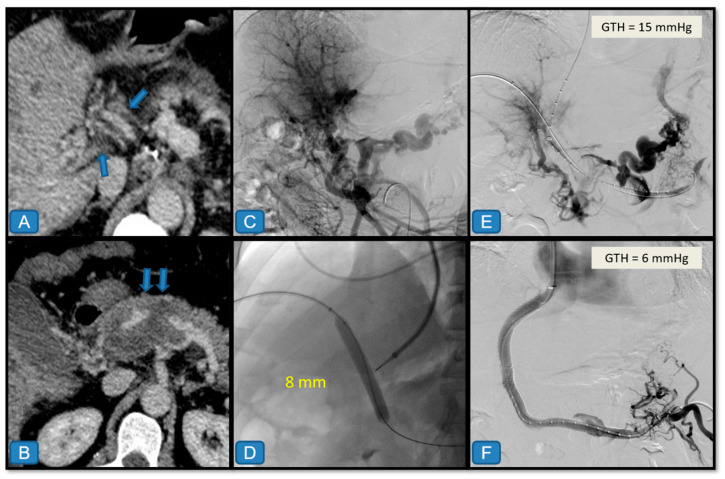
Combined transjugular and transhepatic approach in a 55-year-old man with liver cirrhosis of unknown etiology and bleeding from gastroesophageal varices. (A,B) Contrast-enhanced computed tomography (portal venous phase) shows chronic portal thrombosis of the main portal vein and splenoportal axis (arrows), with features of cavernomatosis. (C) Indirect portography shows a chronic-appearing thrombosis of the portal vein trunk with extensive periportal collateral circulation (cavernomatosis) that recanalizes intrahepatic portal branches. (D) Ultrasound-guided percutaneous transhepatic puncture was performed with a 21 G needle to access a right portal branch. A 6 F introducer sheath was then placed, through which a 5 F catheter and a 0.035″ hydrophilic guide were introduced, achieving recanalization of the portal occlusion through angioplasty with a conventional 8 mm balloon. Subsequently, through transjugular access and using the balloon as a target to reach the portal vein from the middle hepatic vein, it was punctured with a 16 G needle to access the portal-splenic system (E). The transhepatic gradient (GTH) was 15 mmHg. (F) A TIPS was created using an ePTFE-covered stent, with an additional uncoated stent extension into the splenic vein. Both stents were remodeled with an 8 mm balloon, achieving a final GTH of 6 mmHg. Finally, embolization of the transhepatic tract was performed using Gelfoam.
